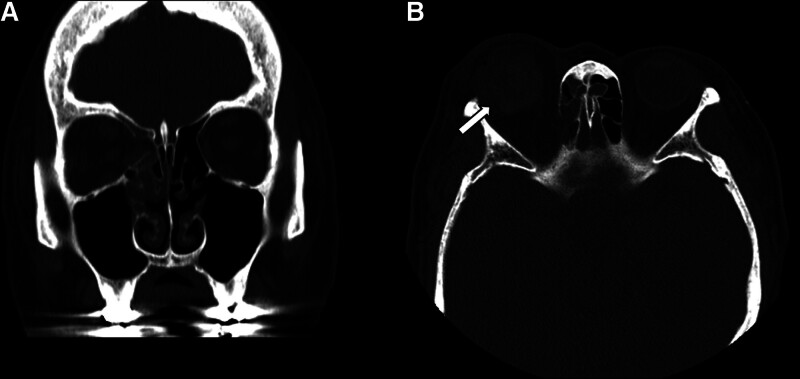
Preoperative computed tomography of the orbit. (A) Coronal view showing a medial orbital wall fracture. (B) Axial view demonstrating the site of posterior temporal scleral rupture with suspected rolled margin (arrow).
